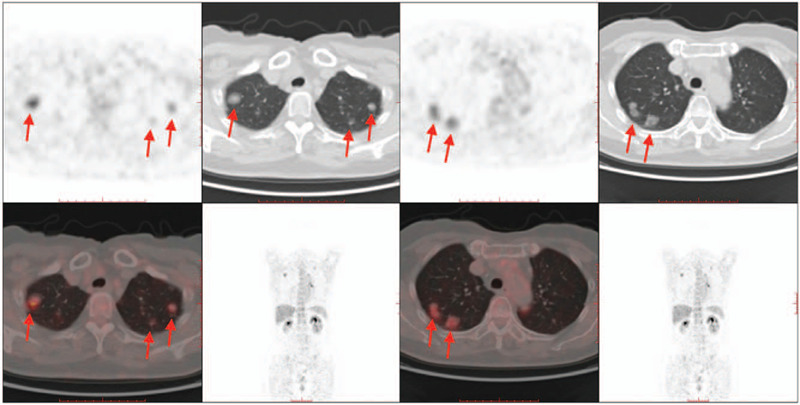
PET-CT demonstrated multiple lung-grinding glass nodules with a partial metabolic increase.
